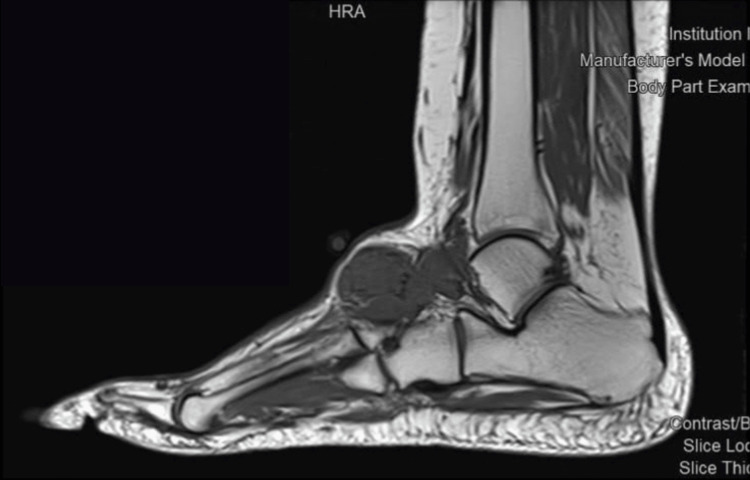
Preoperative magnetic resonance imaging study of the left foot lateral view showing heterogeneous lobulated soft tissue mass at the superior aspect of the tarsal midfoot bones.
